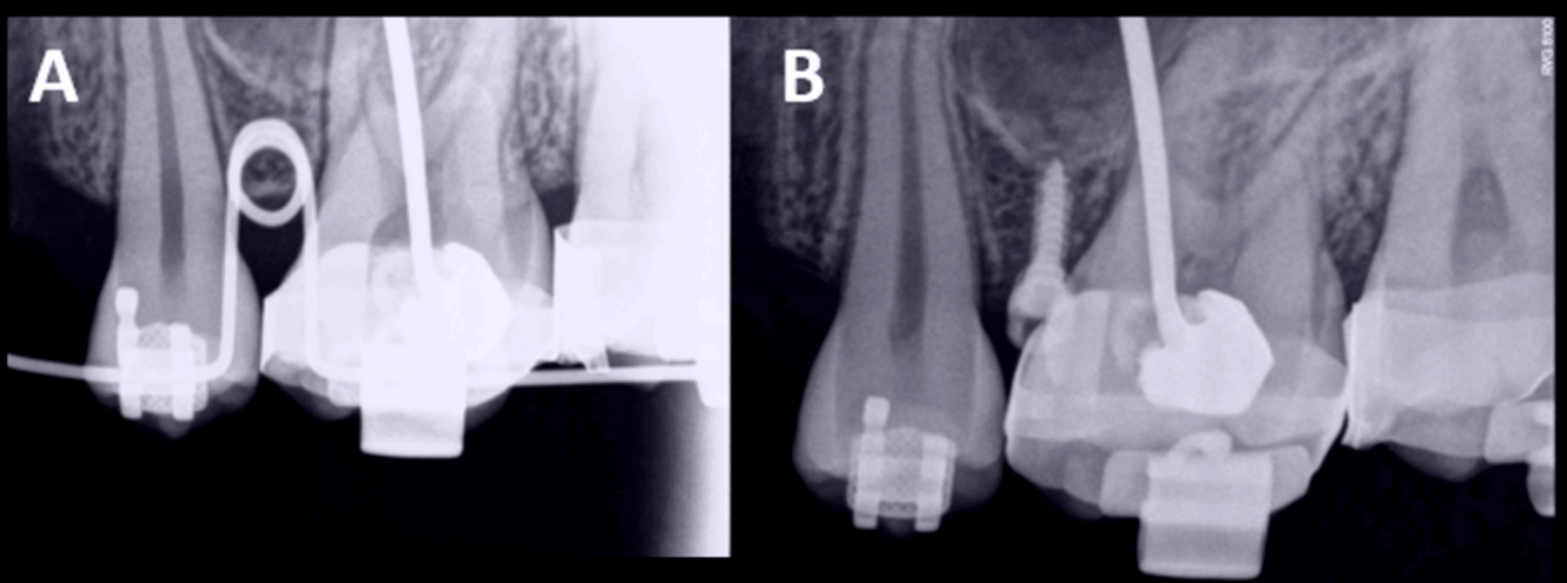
(A) IOPA radiograph to ensure interradicular position of the mini-implant placement jig. (B) IOPA radiograph after mini-implant placement in the interradicular bone between the second premolar and first molar IOPA: intraoral periapical.
